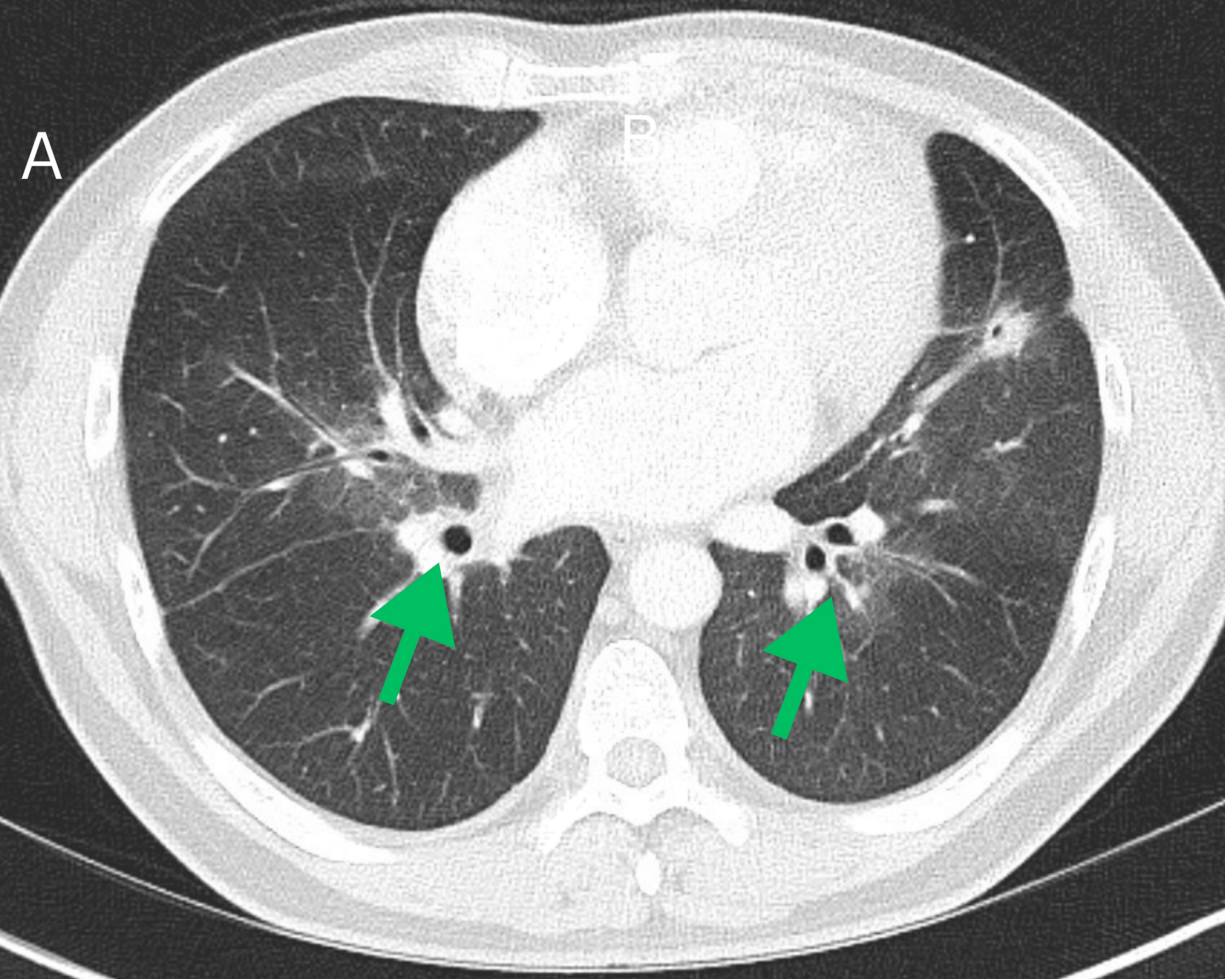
CT chest obtained at the two-month follow-up shows continued improvement in the primary tumor and lymphadenopathy, with an overall decrease in disease burden.
